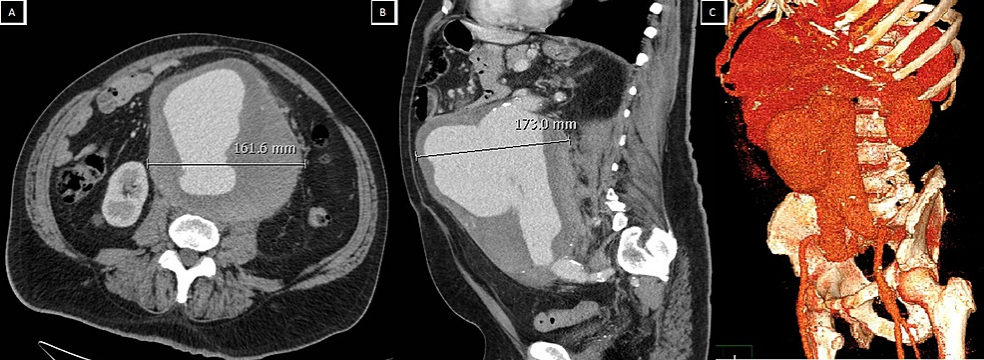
CT-angiogram demonstrating the infra-renal abdominal aortic aneurysm prior to surgery in axial (A), sagittal (B), and reconstruction (left oblique) (C) views.
